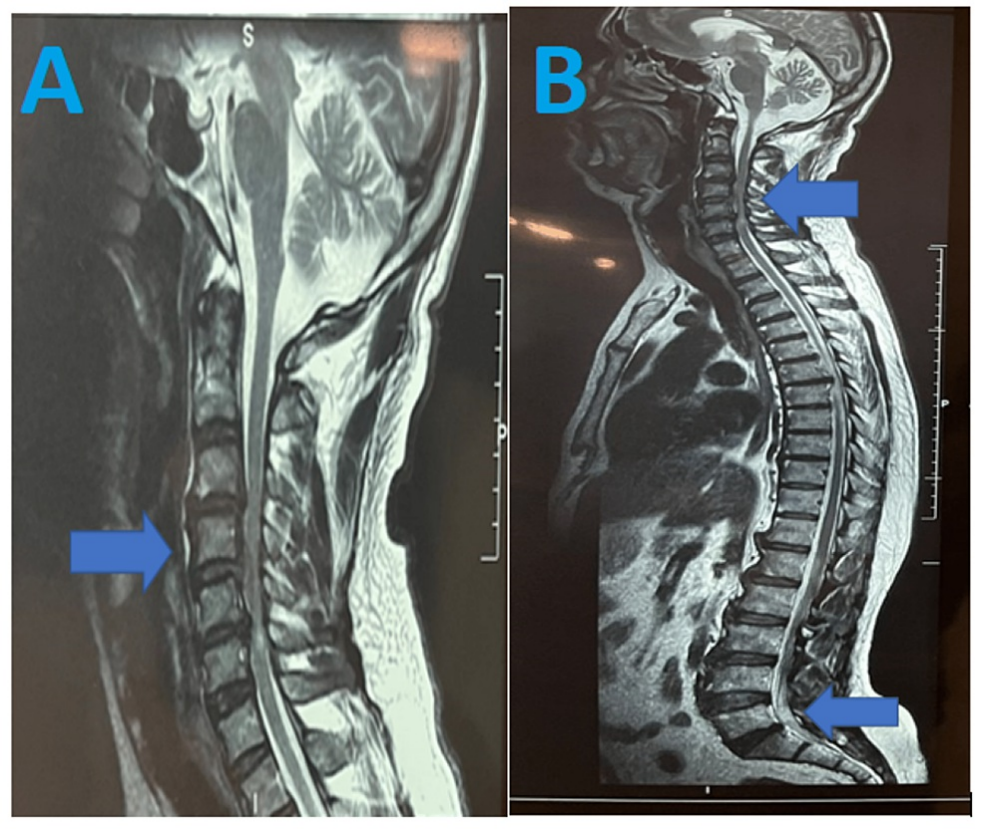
MRI of the cervical spine (A) The blue arrow indicates multiple levels (C3-C6) where diffuse disc bulge indenting over the thecal sac is seen. (B) MRI of the full length of the spine. The upper blue arrow shows a disc bulge at the cervical spine and the lower blue arrow is indicative of a disc bulge between the fifth lumbar and first sacral vertebrae MRI: magnetic resonance imaging
